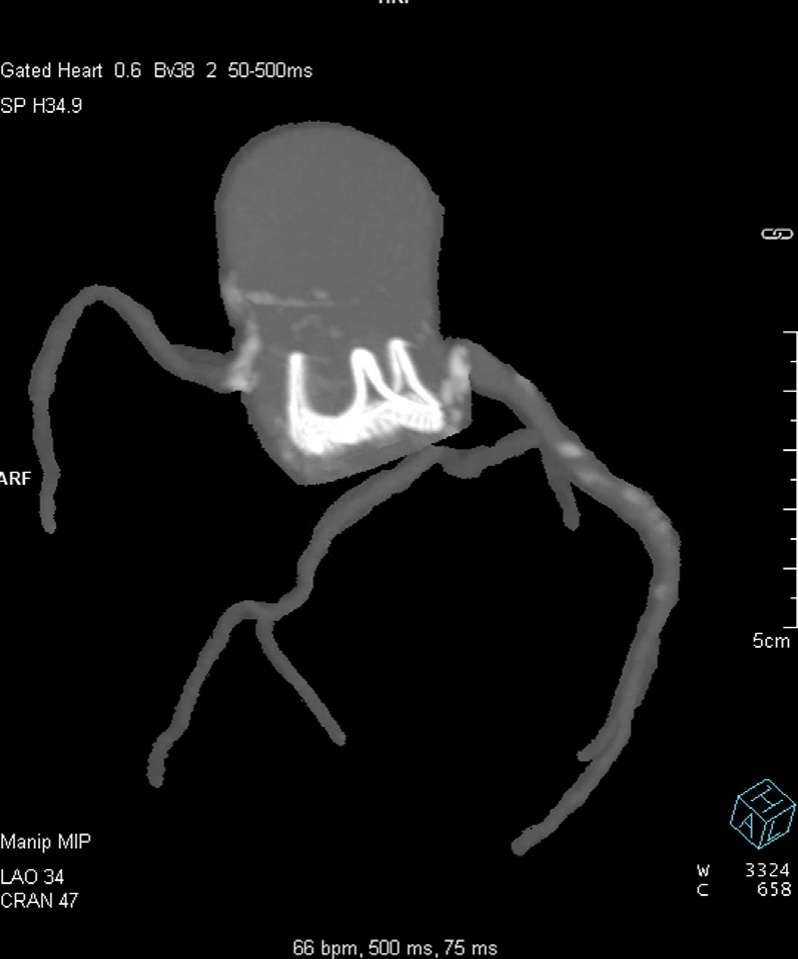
Transcatheter Aortic Valve Implantation Planning CT Scan Demonstrating Perimount Implant With Severe Calcification Abutting Left Main Stem
